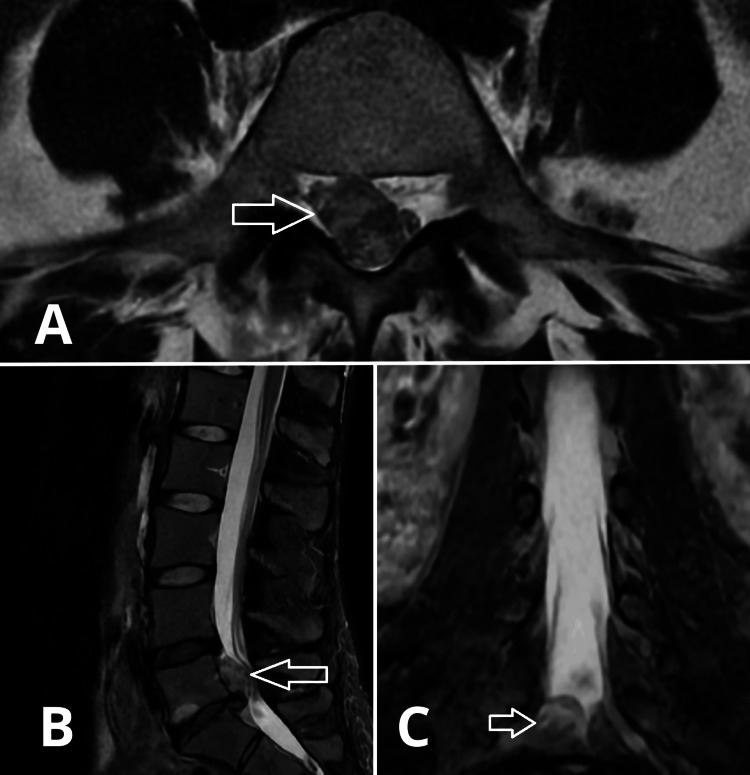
Pre-operative lumbar spine MRI scan revealed a large right-sided cranially migrated L5-S1 lumbar disc herniation (showed by a white arrow). A) Axial view. B) Sagittal view. C) Coronal view.
